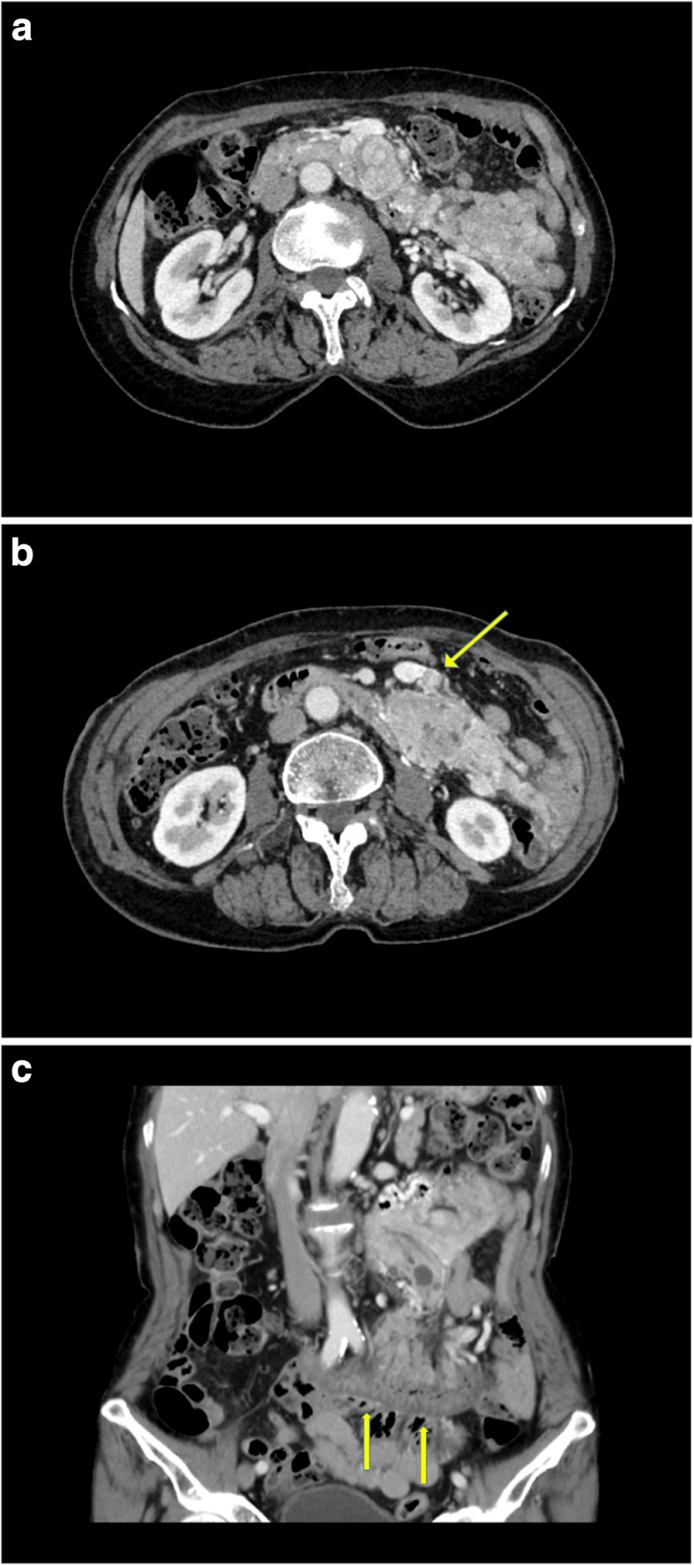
Preoperative computed tomography scans: a. An 8.5 × 4.0 cm exophytic submucosal tumor located in the jejunum. The posterior aspect of the superior mesenteric vein is compressed by the tumor, and the tumor extends to the uncinate process of the pancreas; b. The tumor thrombus invades the first jejunal vein (*arrow*); c. The jejunal mesentery shows significant thickening (*arrow*)
